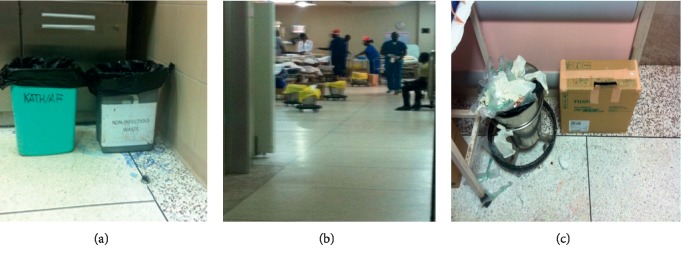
Waste sorting at various units of KATH. (a) Hematology unit. (b) Resuscitation unit. (c) Radiography unit.
